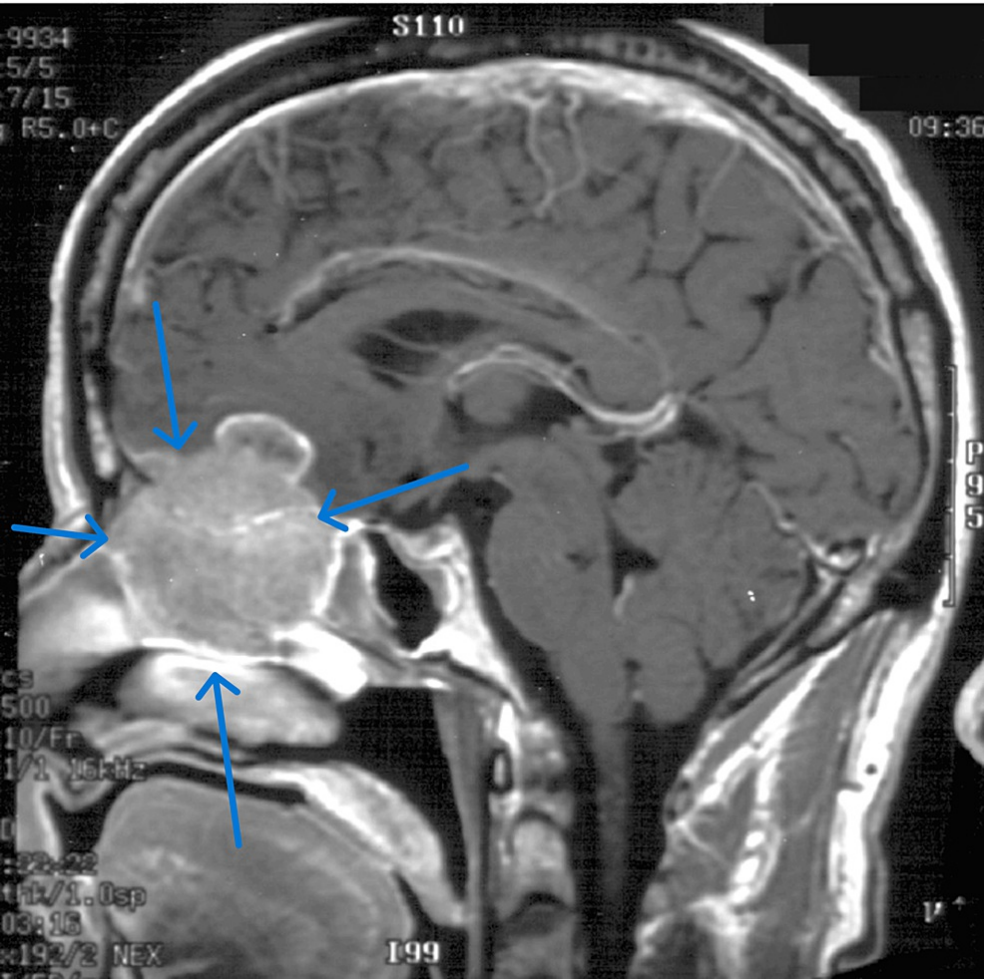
MRI sagittal section scan with contrast of olfactory neuroblastoma extends cephalad to anterior cranial fossa through the cribriform plate
